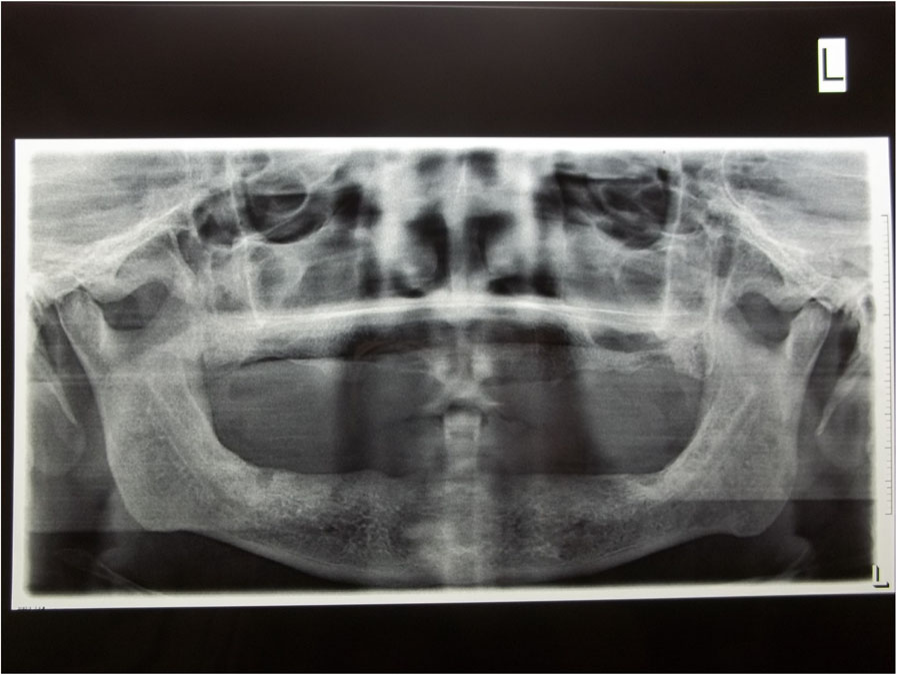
Panoramic radiograph shows no osseous deformities seen in maxilla and mandible
